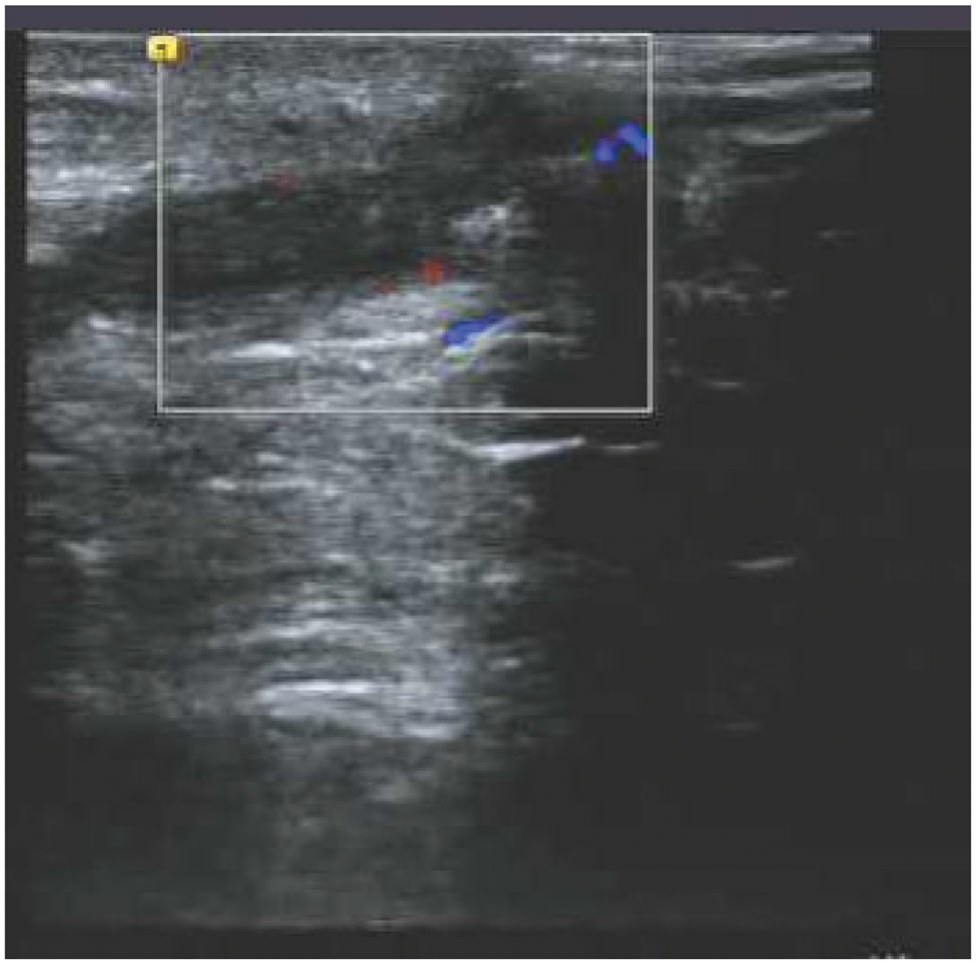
B-Mode Ultrasound Image of case 2 (a).
